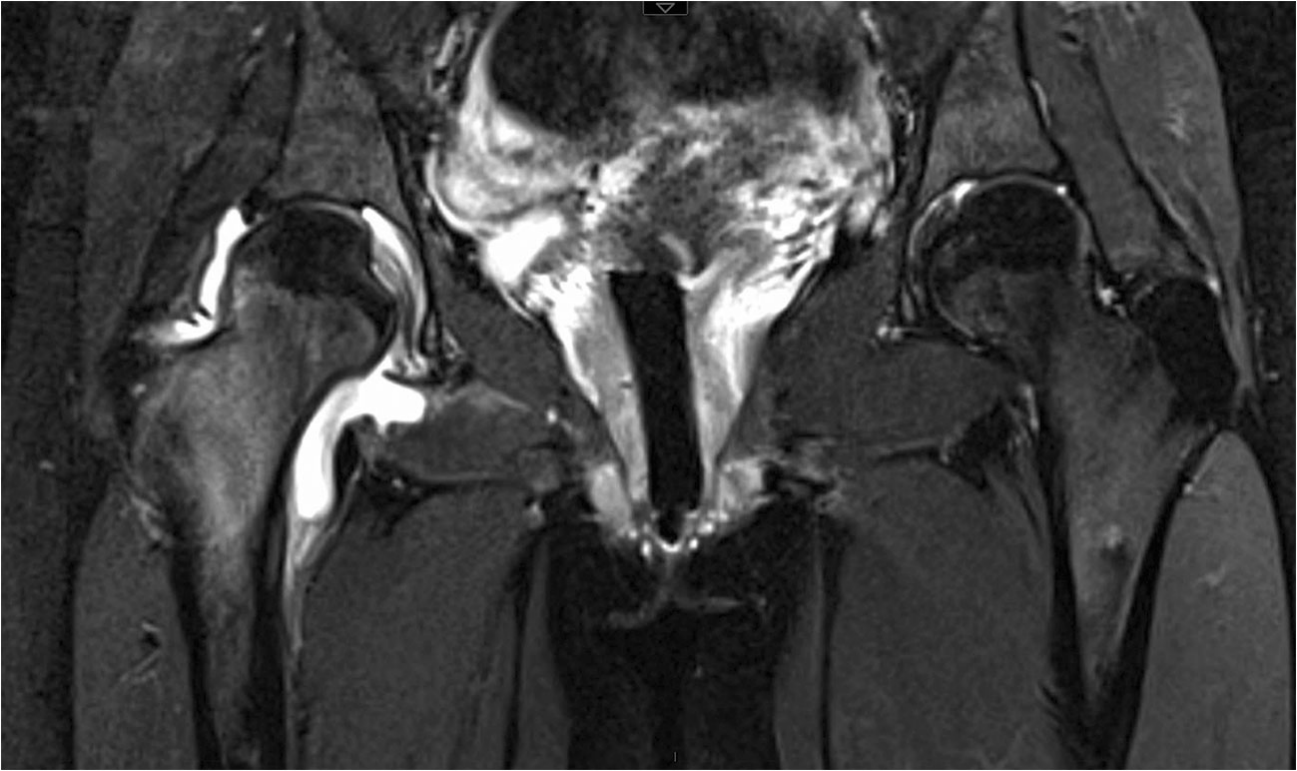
OO mimicker of arthritis. Coronal fat-saturated T2-weighted MR images of the pelvis shows synovial thickening and edema-like bone marrow in the right femoral neck. Hip arthritis had been diagnosed and treated with local steroid injections. A bone scintigraphy suggested a diagnosis of OO at the anterior aspect of the femoral neck, confirmed by a CT scan (not shown)
